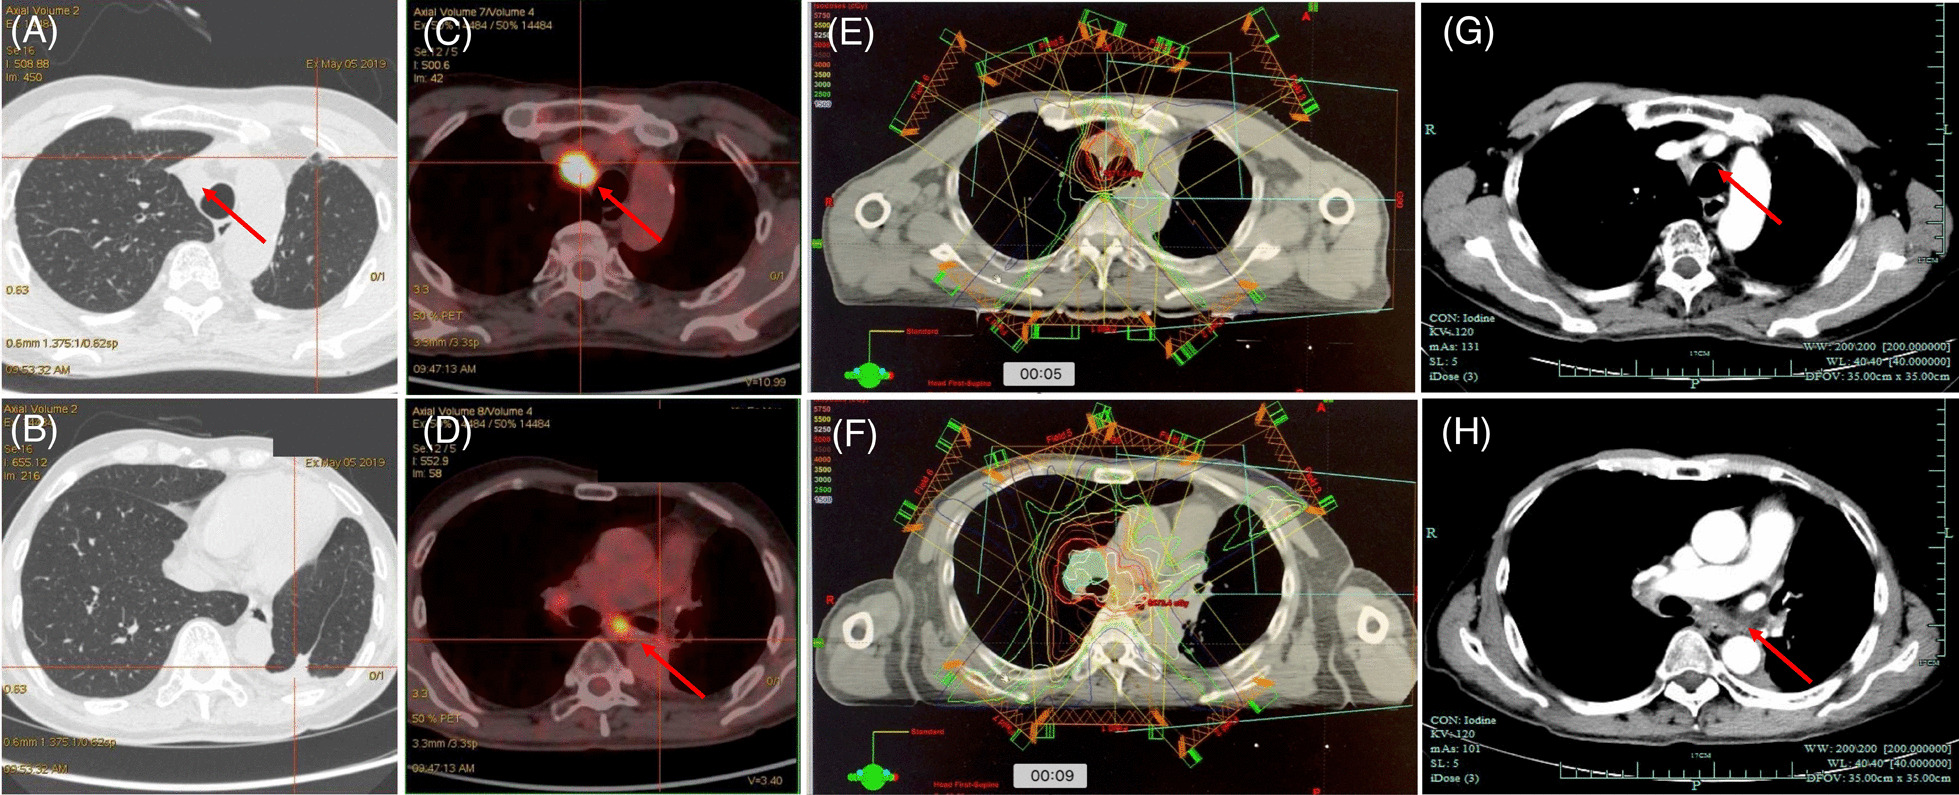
A–D PET-CT scan capture on May 5th, 2019. E, F Radiation dosimetry of re-irradiation; G, H CT lung and contrast window capture on October 29th, 2019 (after re-irradiation). Significant abnormal findings noted (arrow)
